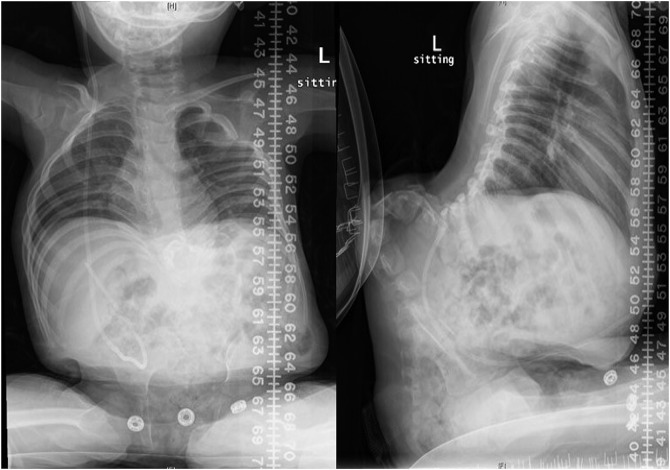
AP and lateral radiographs of the thoracolumbar kyphotic deformity. The posterior spinal elements are deficient at the periapical region. The apex of the gibbus is at L2.
